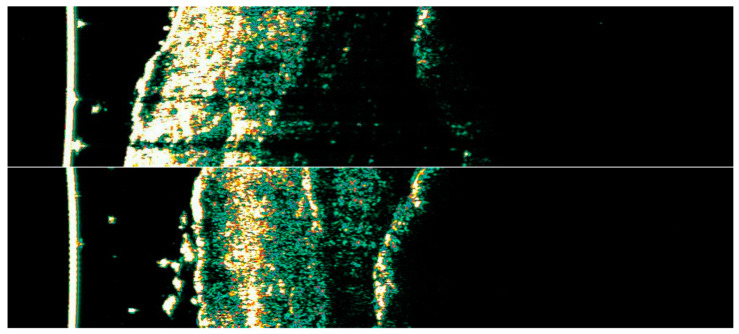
Ultrasonographic images of BCC on the temple.
